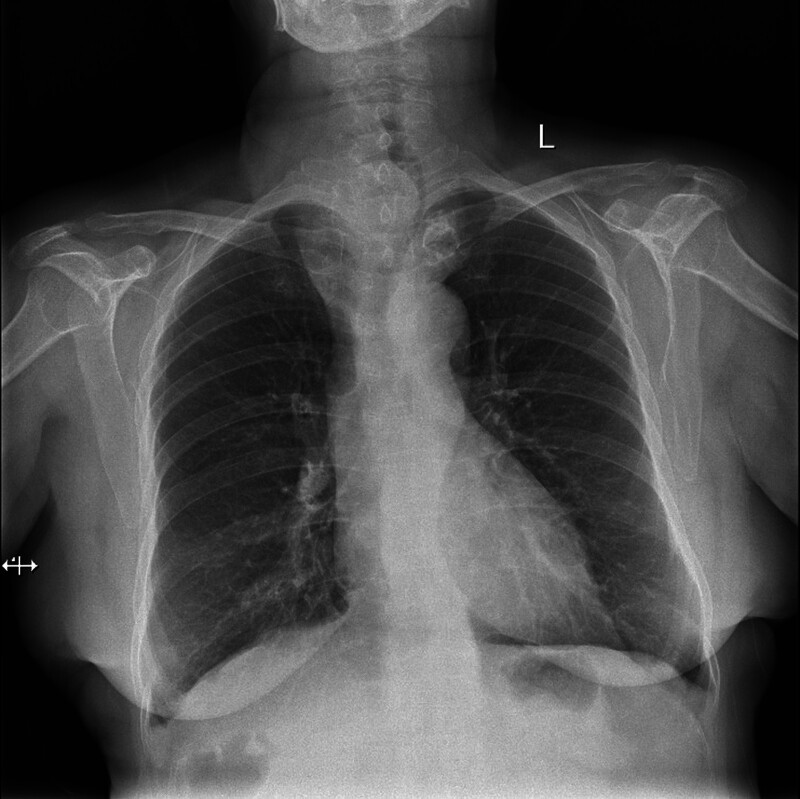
Chest X-ray: quasi-circular iso-hyper-density lesions can be seen on the right side of the upper mediastinum, and the airway is pushed to the left due to airway compression.
